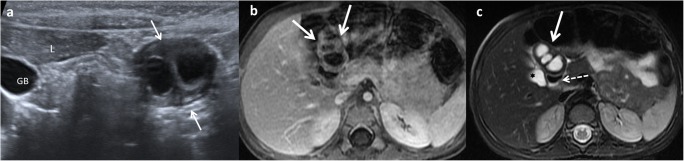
A 2-year-old girl with fever and abdominal pain. a Abdominal US view of a complex cystic lesion (*white arrows*) in the duodenal area, next to the liver (*L*). *GB* gallbladder. b Axial gadolinium-enhanced GRE T1 demonstrating the presence of a multilocular cystic mass (*white arrows*) with wall thickening. c Axial T2 MRI: the mass (*white arrow*) shows three cystic cavities inside, close to the anterior wall of the duodenum (*dashed-line arrow*) and lateral to the gallbladder (*star*). Surgical findings: multiple duodenal duplication cyst, without connection with the lumen and with ectopic gastric mucosa
